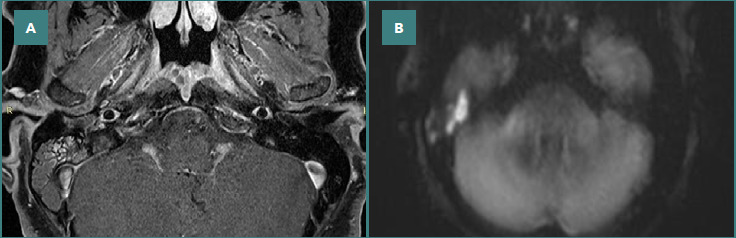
Magnetic resonance imaging findings of cholesteatoma and associated inflammatory changes. A, Axial non-EPI DWI showing restricted diffusion (high signal) in the deep EAC, tympanic cavity, and mastoid antrum, confirming cholesteatoma; the mastoid air cells lacked restricted diffusion, establishing clear compartmentalization. B, Axial T1-weighted postgadolinium sequence showing absent enhancement in the tympanic cavity, distinguishing cholesteatoma from granulation tissue; moderate enhancement of the external auditory canal wall reflects chronic otitis externa.
